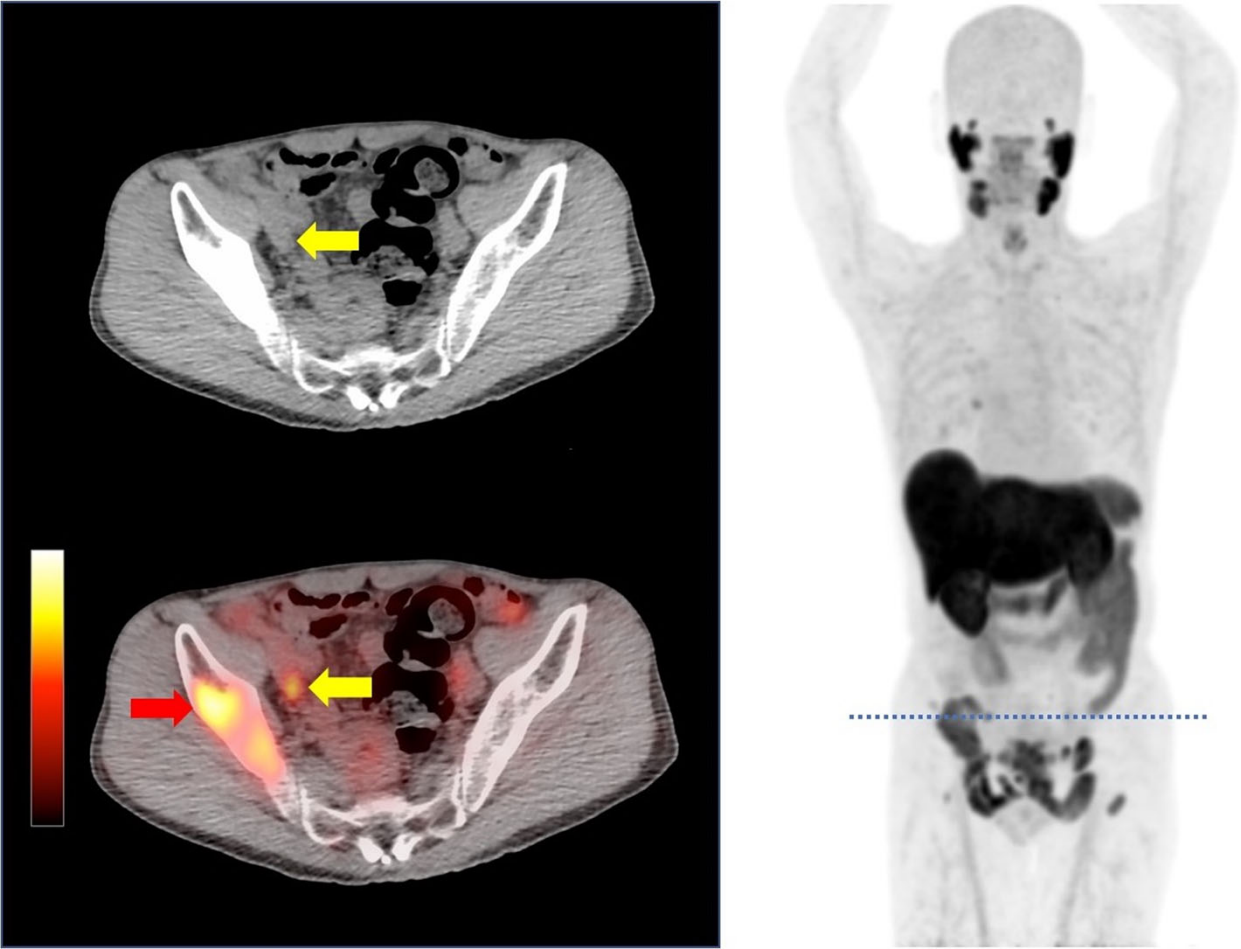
[^18^F]PSMA-1007 PET/CT images of a metastatic prostate cancer patient. A dotted cross section of whole-body PET (right) is displayed on the CT and PET/CT image (left). In addition to right iliac metastasis (red arrow), we observed a small lymph node metastatic lesion (yellow arrows), which would be virtually impossible to detect by conventional CT imaging
